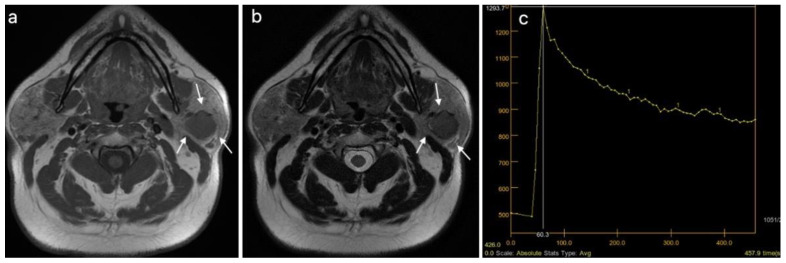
Warthin tumor of the left parotid gland (white arrows): (a) T1WI sequence showing a hypointense signal in the tumor, well delineated, with slightly irregular borders; (b) T2WI sequence showing a predominantly hypointense signal in the lesion, with peripheral cystic area; (c) DCE-MRI showing a type B TIC curve.
